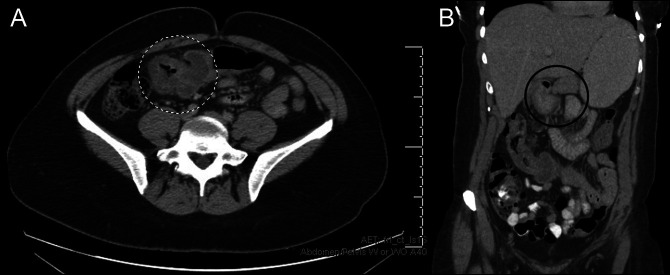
(A and B) Initial abdominal and pelvic computed tomography with contrast showed signs of intussusception of the transverse colon near the hepatic flexure.
